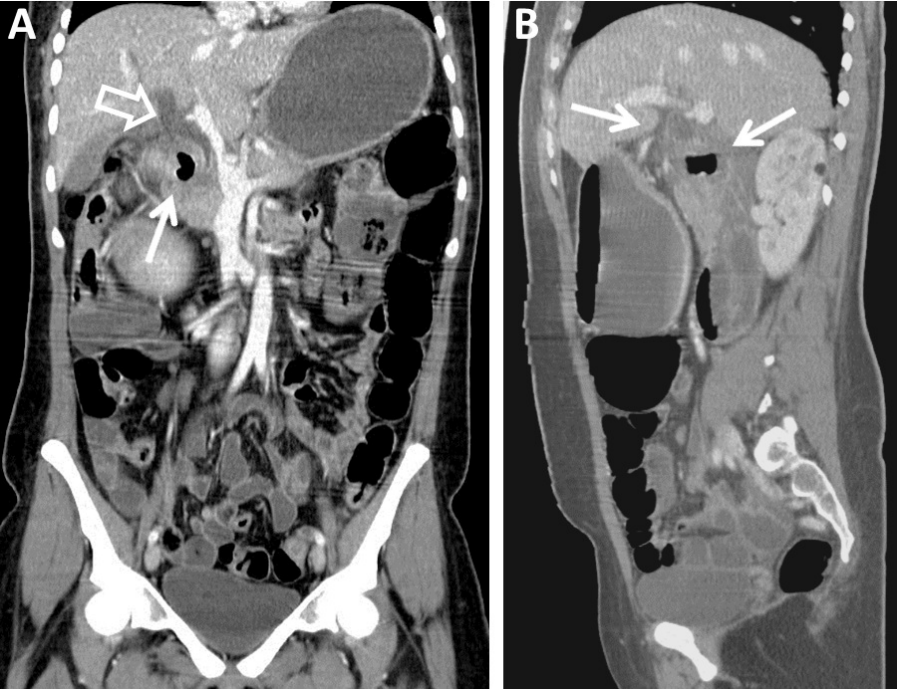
Contrast-enhanced Coronal and Sagittal CT of the Abdomen and Pelvis (Pre-Treatment) Contrast-enhanced reformatted images of the abdomen and pelvis demonstrate (A) an inflamed periampullary diverticulum, which obstructs the common bile duct (arrow). The common bile duct (open arrow) is significantly dilated. In addition, intrahepatic biliary ductal dilation is seen. (B) The sagittal image provides another view of the duodenal diverticulitis and common bile duct dilation (arrows).
